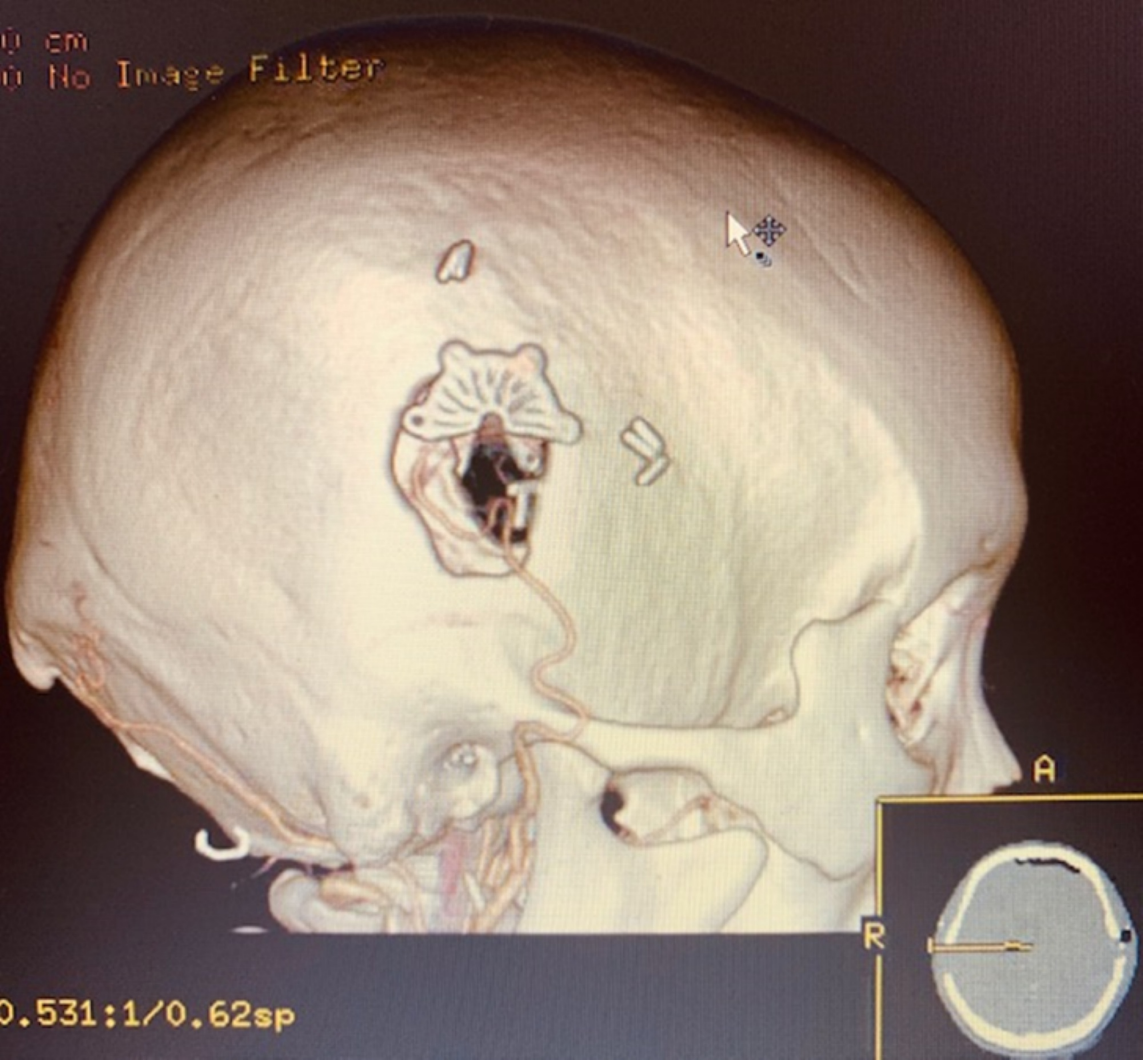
Immediate postoperative CT angiogram confirming direct bypass anastomosis through a minimally invasive approach
